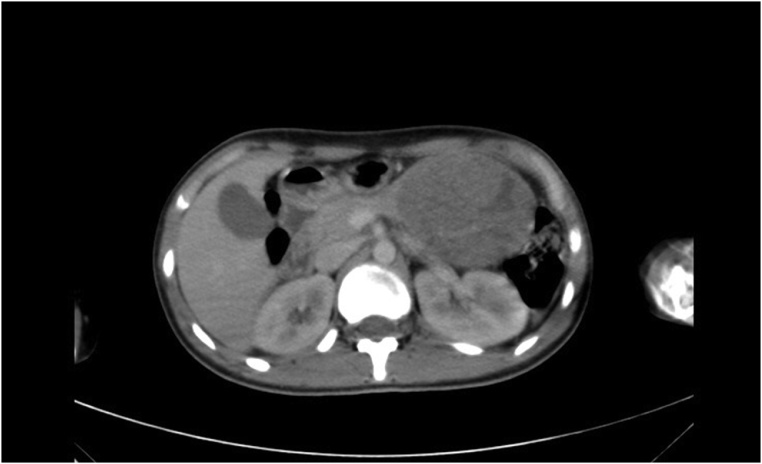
Computed tomography scan of the abdomen showing a mass in the region of the tail of the pancreas.
